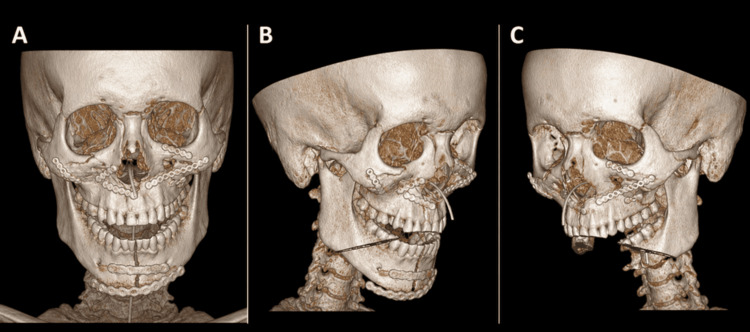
Postoperative volume-rendered CT images show (A) a frontal view, (B) a left oblique/lateral view, and (C) a right oblique/lateral view. The images demonstrate stable fixation of the midface and mandible, with maintained alignment and reduction of both mandibular condyles following operative management. CT, computed tomography
